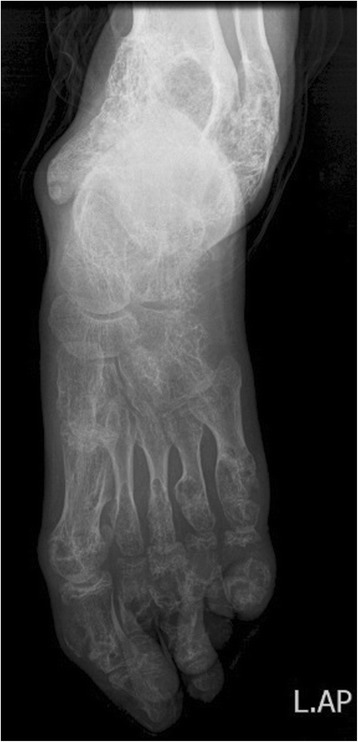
Anterior posterior x-ray of left foot: Multiple expansile lytic lesions in the metatarsi and toes of left foot with bone deformity and lytic lesions in the metaphysis of distal tibia and fibula
